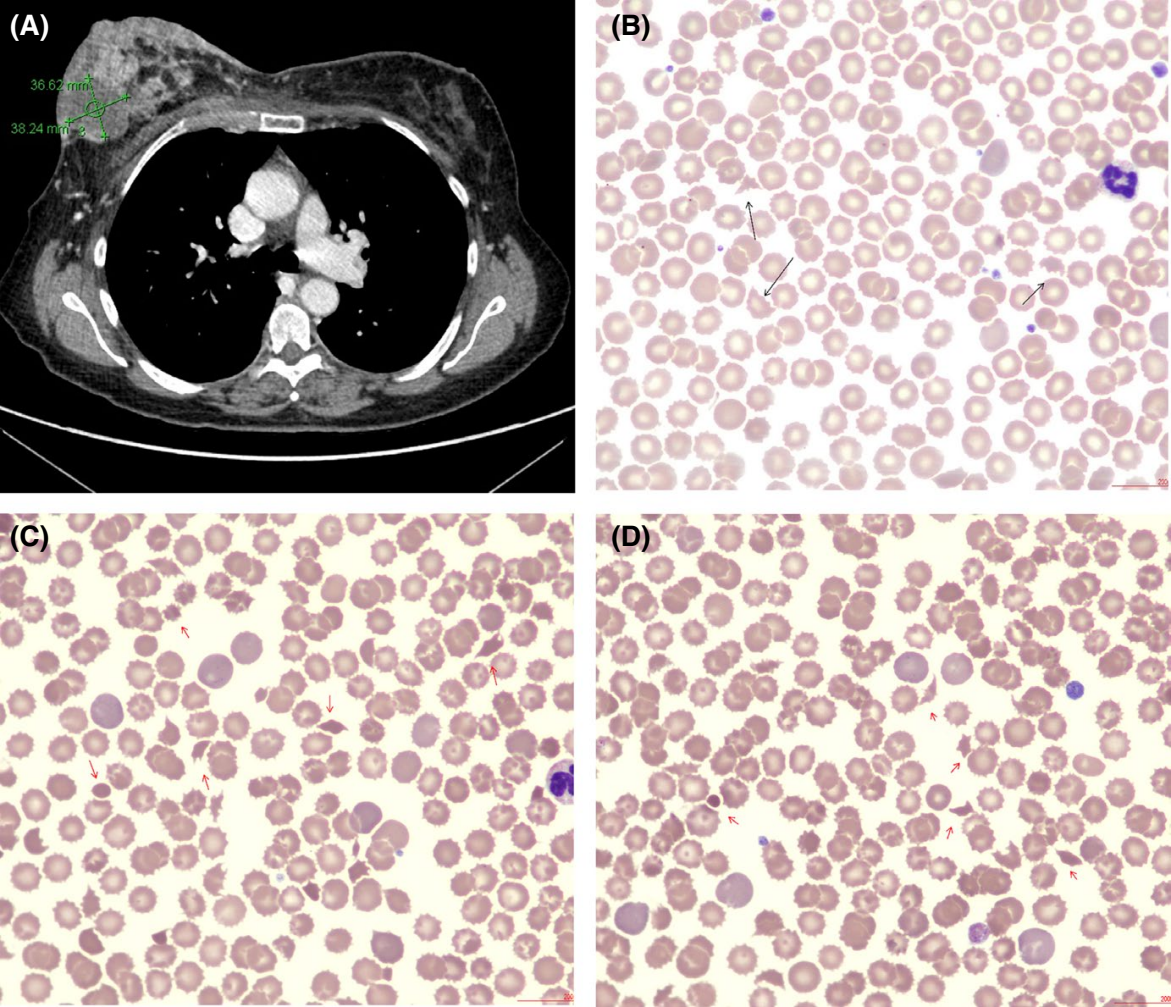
Morphological evidence of brisk cancer‐associated microangiopathic hemolysis is seen. (Panel A) shows the computed tomography image of a right‐sided ulcerating breast mass. At admission on day one (panel B), echinocytes, acanthocytes, and occasional schistocytes are seen. Over the next two days (panels C and D), significant red cell fragmentation developed just prior to patient's demise
